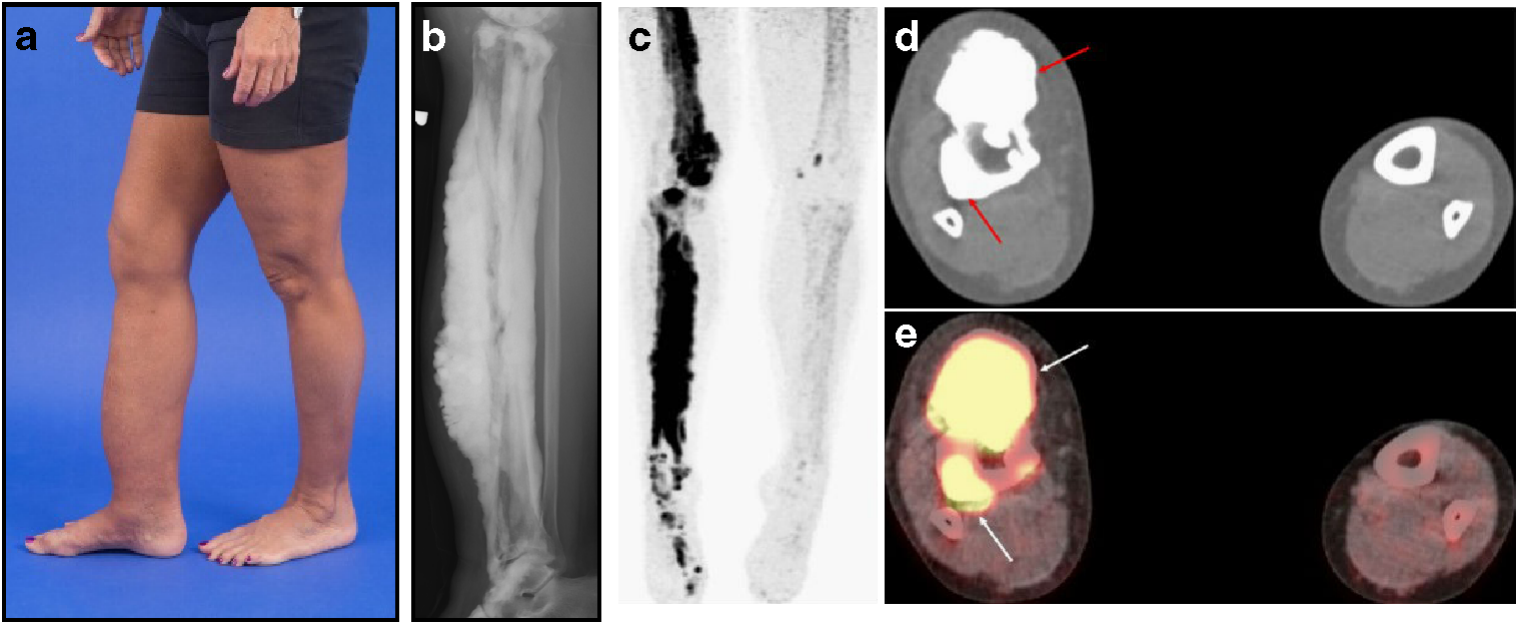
Clinical findings in melorheostosis. a Clinical appearance of Melo-10 with melorheostosis of right lower extremity. Note irregular thickening of the right leg. The affected bone was found to harbor a *MAP2K1* mutation. b Radiograph of the right tibia/fibula of Melo-10 with classic candle-wax appearance. c Maximal intensity projection (MIP) ^18^F-NaF PET image of the lower extremities showing intensely increased ^18^F-NaF activity in the bones of the right leg, primarily along the medial and distal femur, and along the tibia extending into the foot. d, e Axial CT and fused ^18^F-NaF PET/CT images at the level of mid-calf demonstrating dense cortical bone formation in the anterior and posterior aspect of the right tibia (red arrows in d with corresponding abnormally increased ^18^F-NaF uptake (white arrows, in e)
